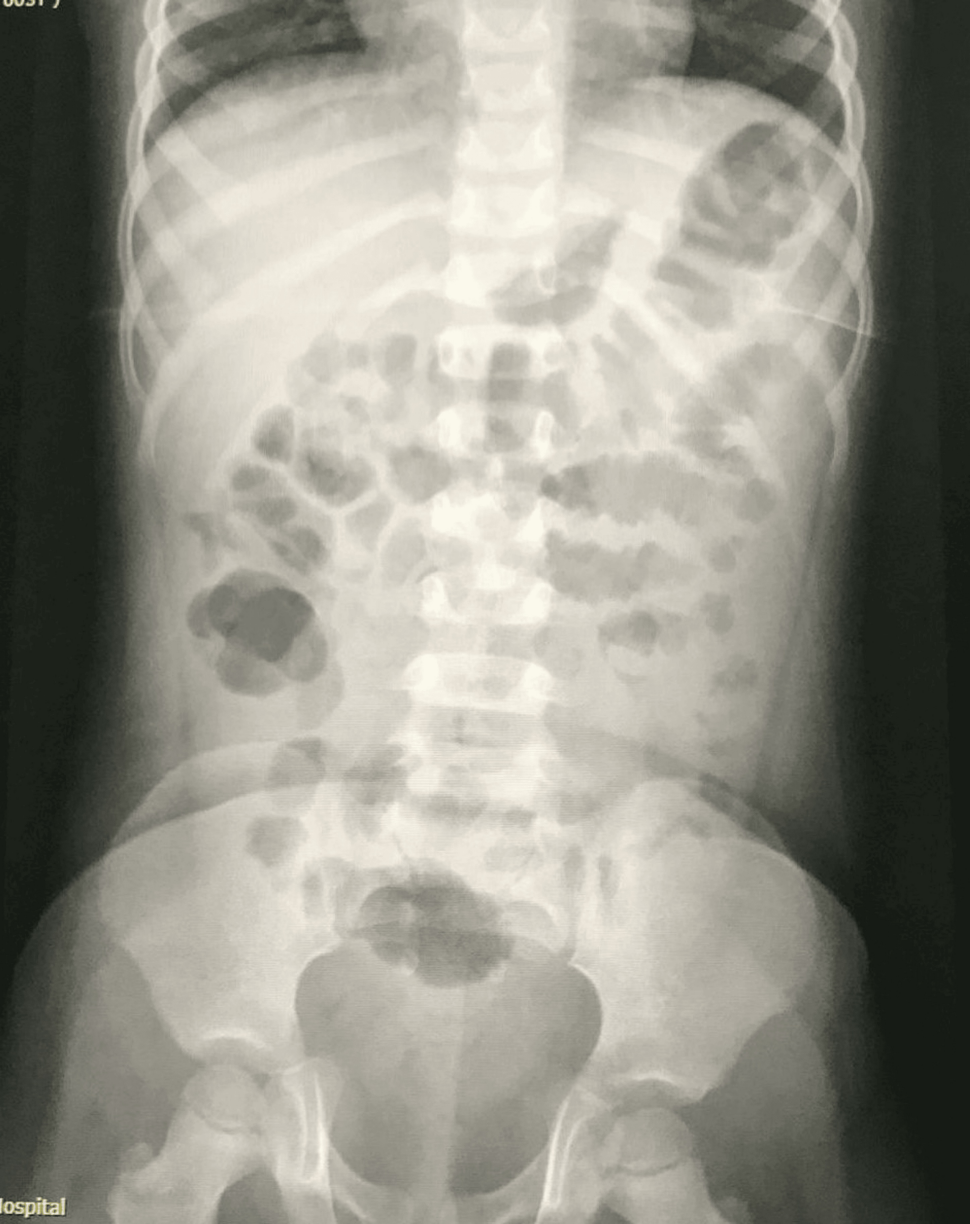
Day 2 normal abdominal X-ray (supine) with normal gas distribution.
